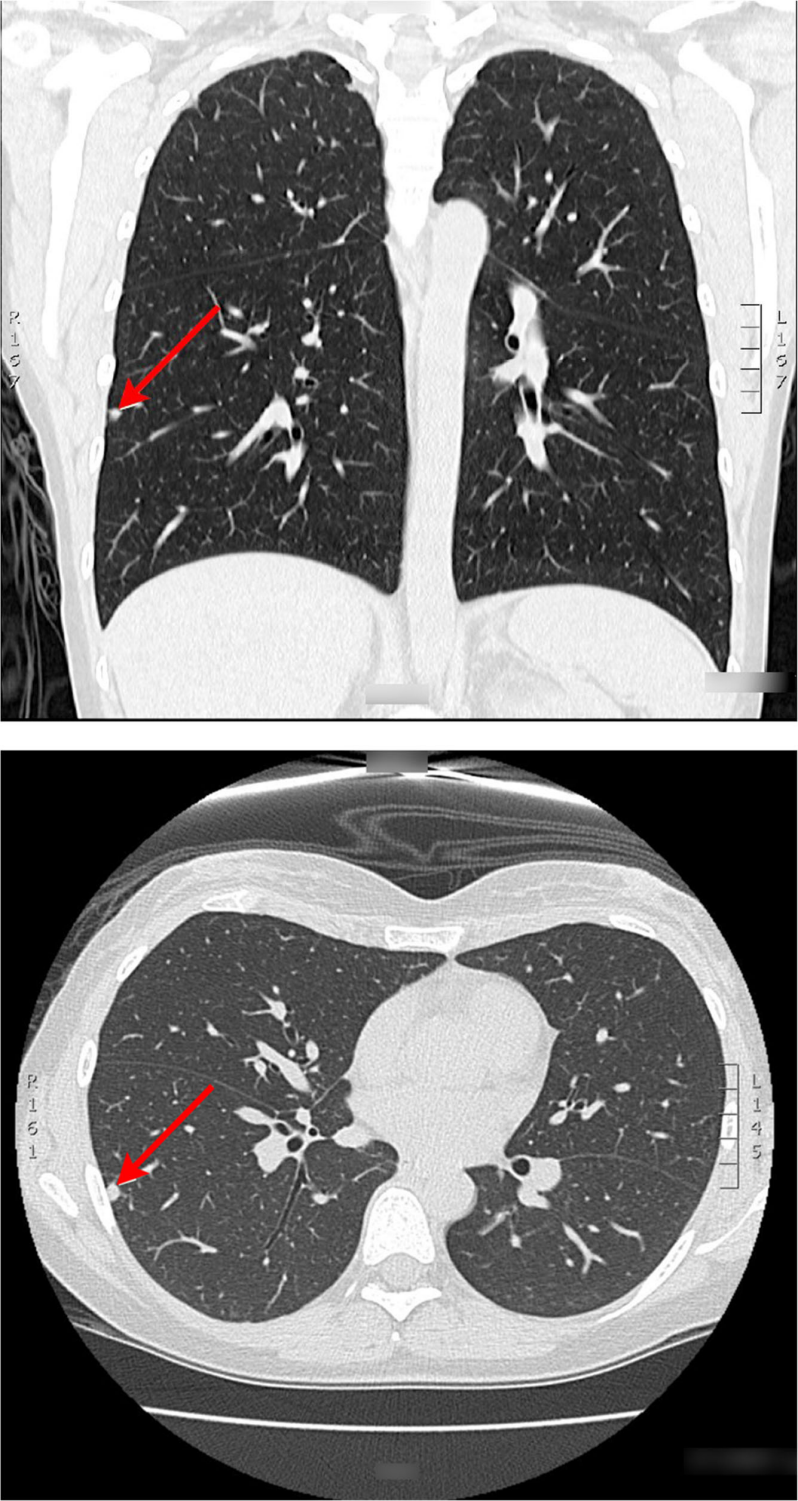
Computed Tomography of Chest, 2013. CT of chest performed in 2013 on first referral to respiratory specialist. CT demonstrates the transient finding of a 6 mm right lower lobe sub pleural nodule, which was transient on serial imaging
